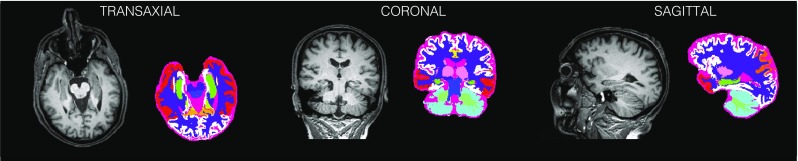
Brain parcellation based on T1w MR image for partial volume correction
